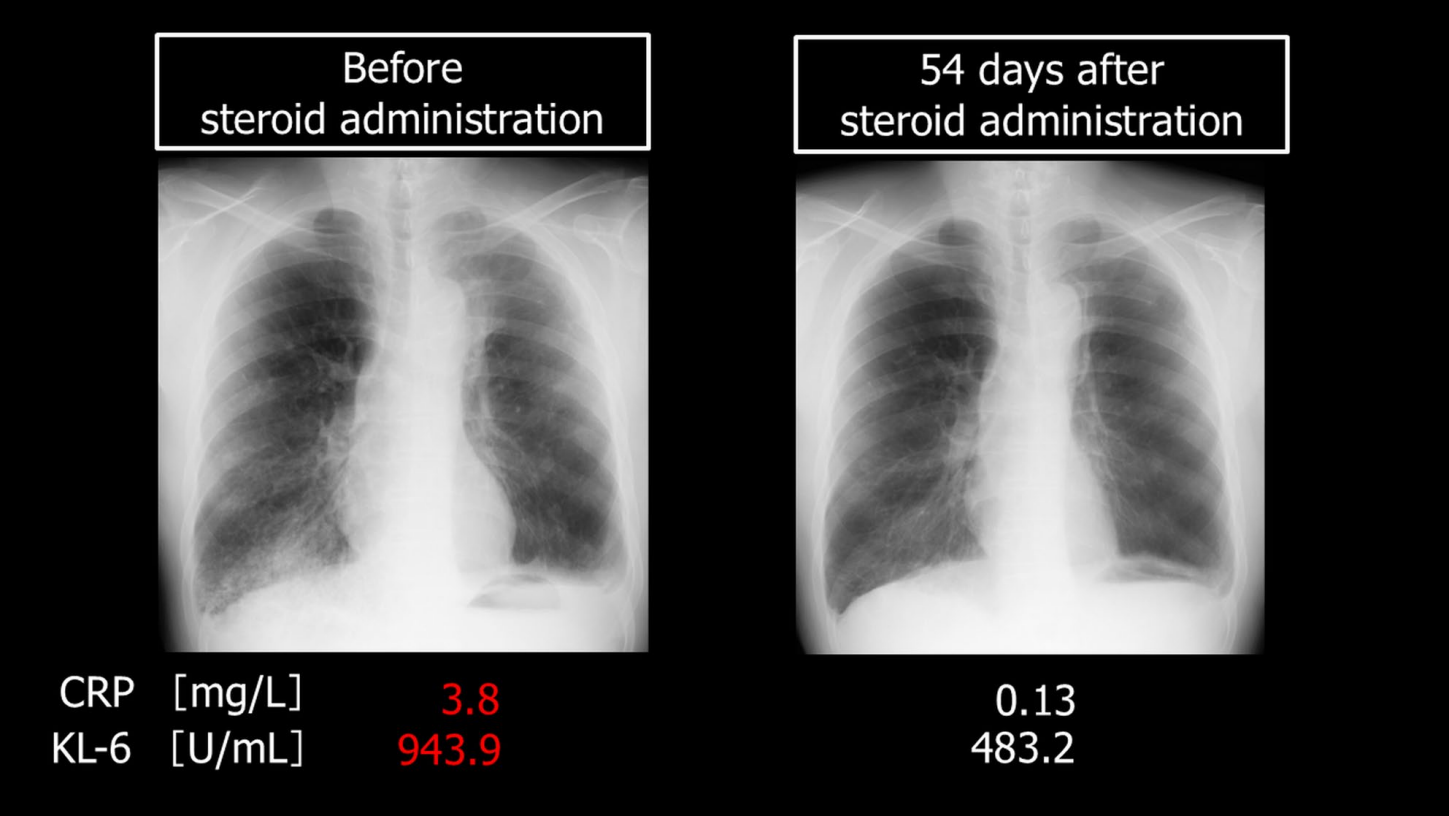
Biochemical test results and chest X-ray imaging before and after steroid administration. This image shows chest X-rays taken before and after steroid administration, along with accompanying biochemical tests. Steroid administration resulted in improvements in areas of decreased permeability in both lower lung fields, as well as an improvement in the inflammatory response and KL-6 levels
